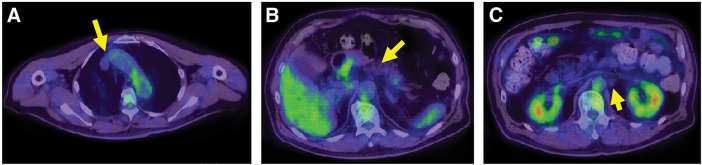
FDG-positron emission-tomography-computed tomography after 6 courses of pembrolizumab therapy. (A) FDG accumulation in the anterior mediastinal lymph node resolved (arrow). (B) FDG accumulation in the primary tumor and surrounding lymph nodes decreased (SUVmax = 1.65) (arrow). (C) FDG accumulation in the para-aortic lymph node resolved (arrow). FDG, ^18^F-fluorodeoxyglucose; SUVmax, maximum standardized uptake value
